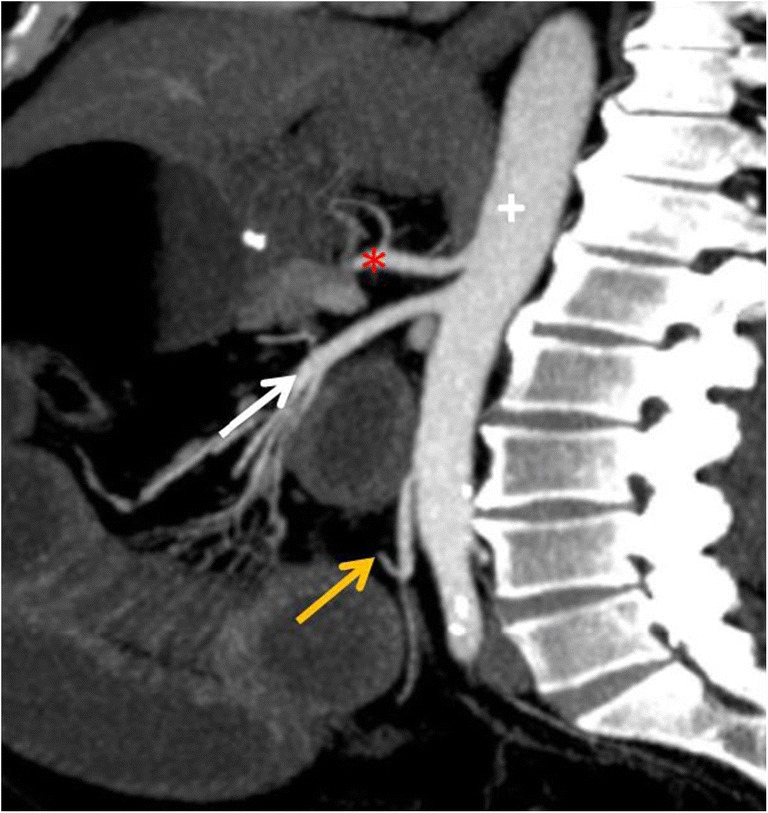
Anatomy. Sagittal CT MIP (maximum intensity projection) image shows three major arteries that supply the bowel, coeliac trunk (asterisk), superior mesenteric artery (white arrow) and inferior mesenteric artery (orange arrow), which are visceral branches of the abdominal aorta (+)
